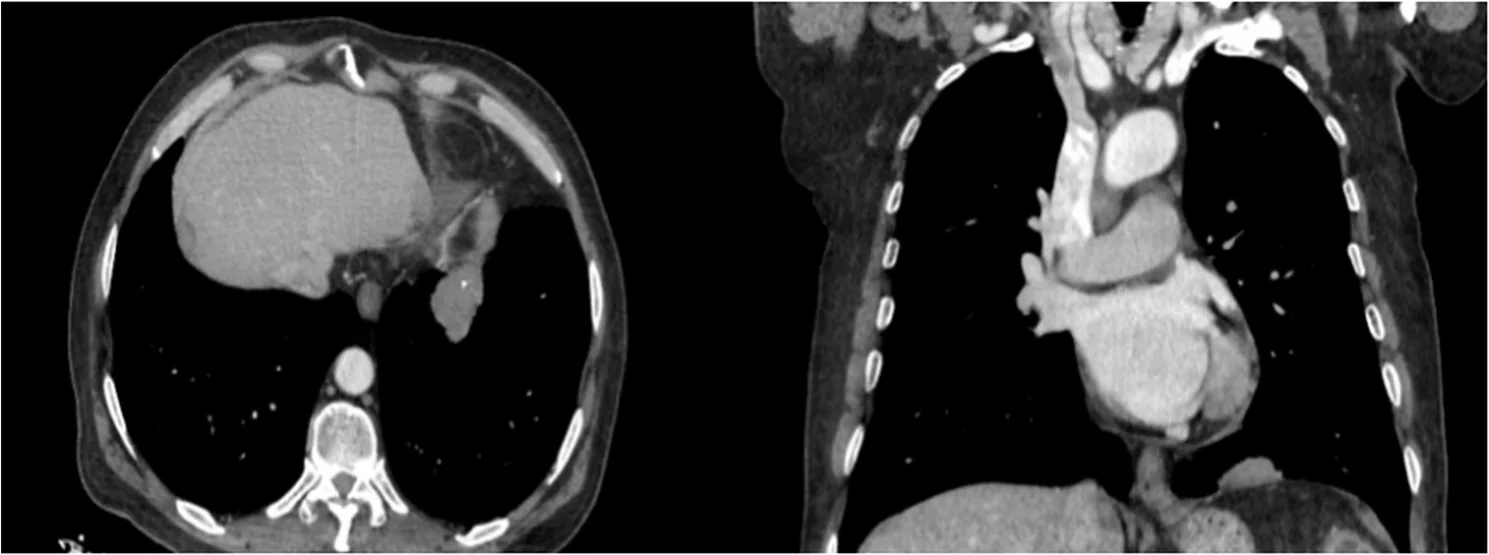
Contrast-enhanced computed tomography showing a well-defined, non-mobile pleural nodule consistent with thoracolithiasis in the left lower hemithorax
